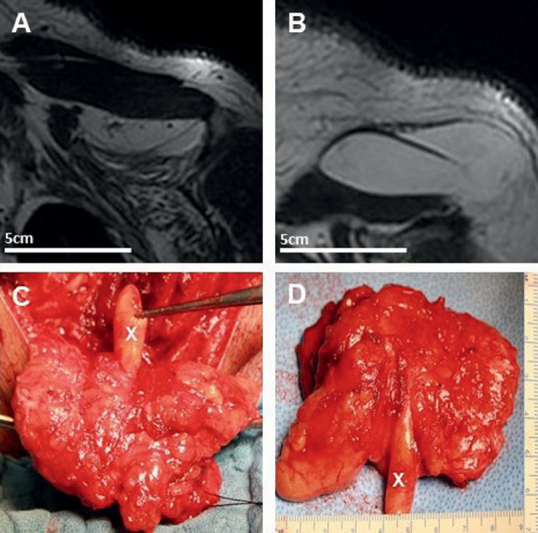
Left-sided brachial plexus lipoma (7 × 9 cm) on T2-weighted fat saturated magnetic resonance imaging (
